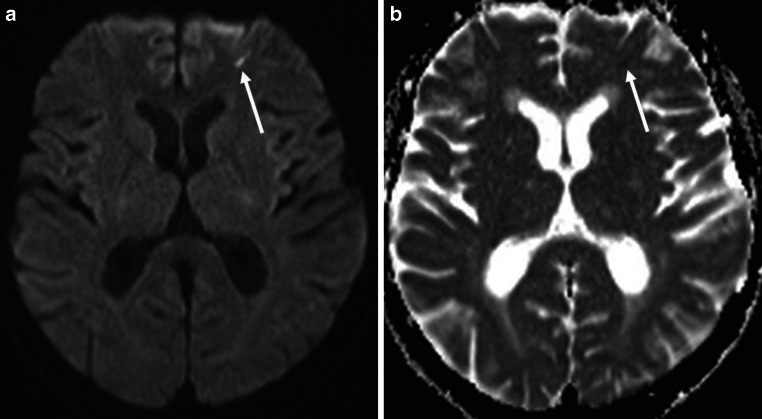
Axial diffusion-weighted imaging (DWI) of the brain with a small DWI lesion (*arrows*) in the left frontal lobe 4 days after angiography. a DWI b-value 1000, b Apparent Diffusion Coefficient (ADC)
